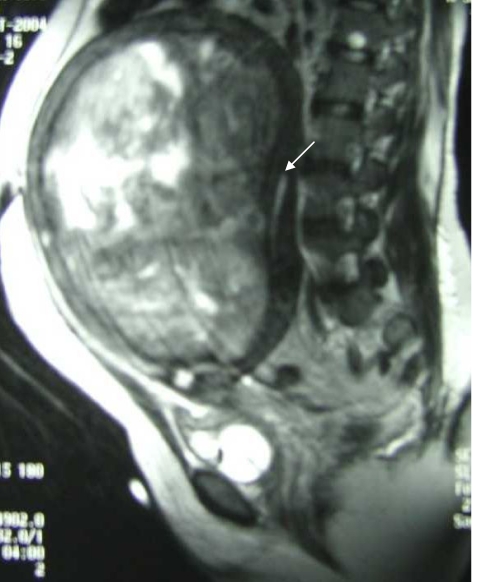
Sagittal T2-weighted section of MRI pelvis demonstrating large low signal intensity mass with multiple areas of high signal intensity within it arising from the anterior uterine wall. The normal endometrial stripe (white arrow) noted with normal thickness, differentiates the mass from gross hydrometra.
